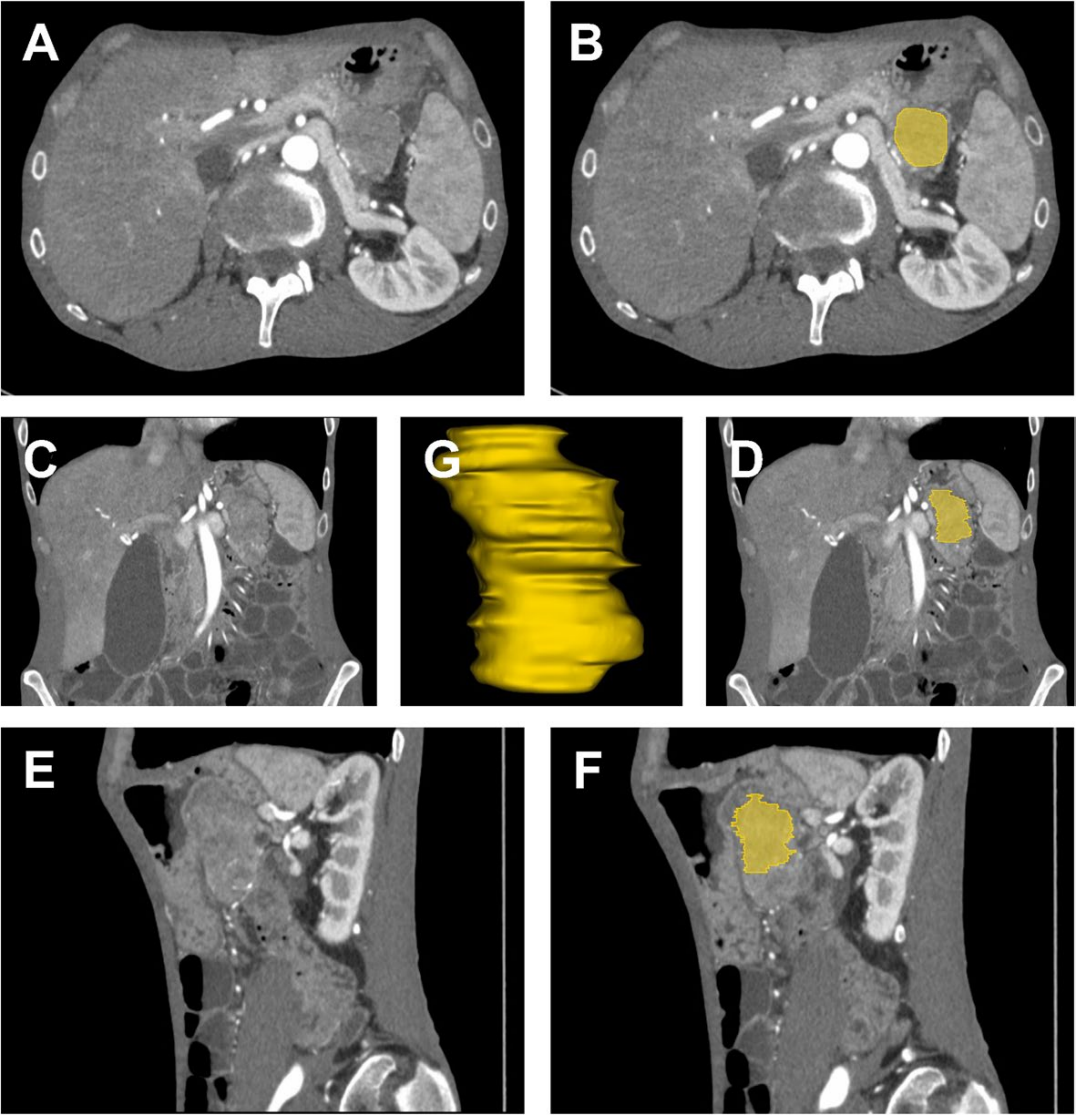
Case example of a 55-year-old male suffering from beltlike abdominal pain with metabolic acidosis (pH 7.3), hypokalemia (2.8 mmol/l), weight loss, and diarrhea for the past three months. After induced infusion therapy to compensate for the electrolyte imbalance, dual-energy computed tomography was performed, showing a hypodense mass of approximately 3.3 × 3.4 × 6.6 cm located in the pancreatic tail with occasional calcifications and finally proven ductal adenocarcinoma (A, C, E). Additionally to tumor segmentation in different planes (B, D, F), the pancreatic mass is also shown in 3D (G)
